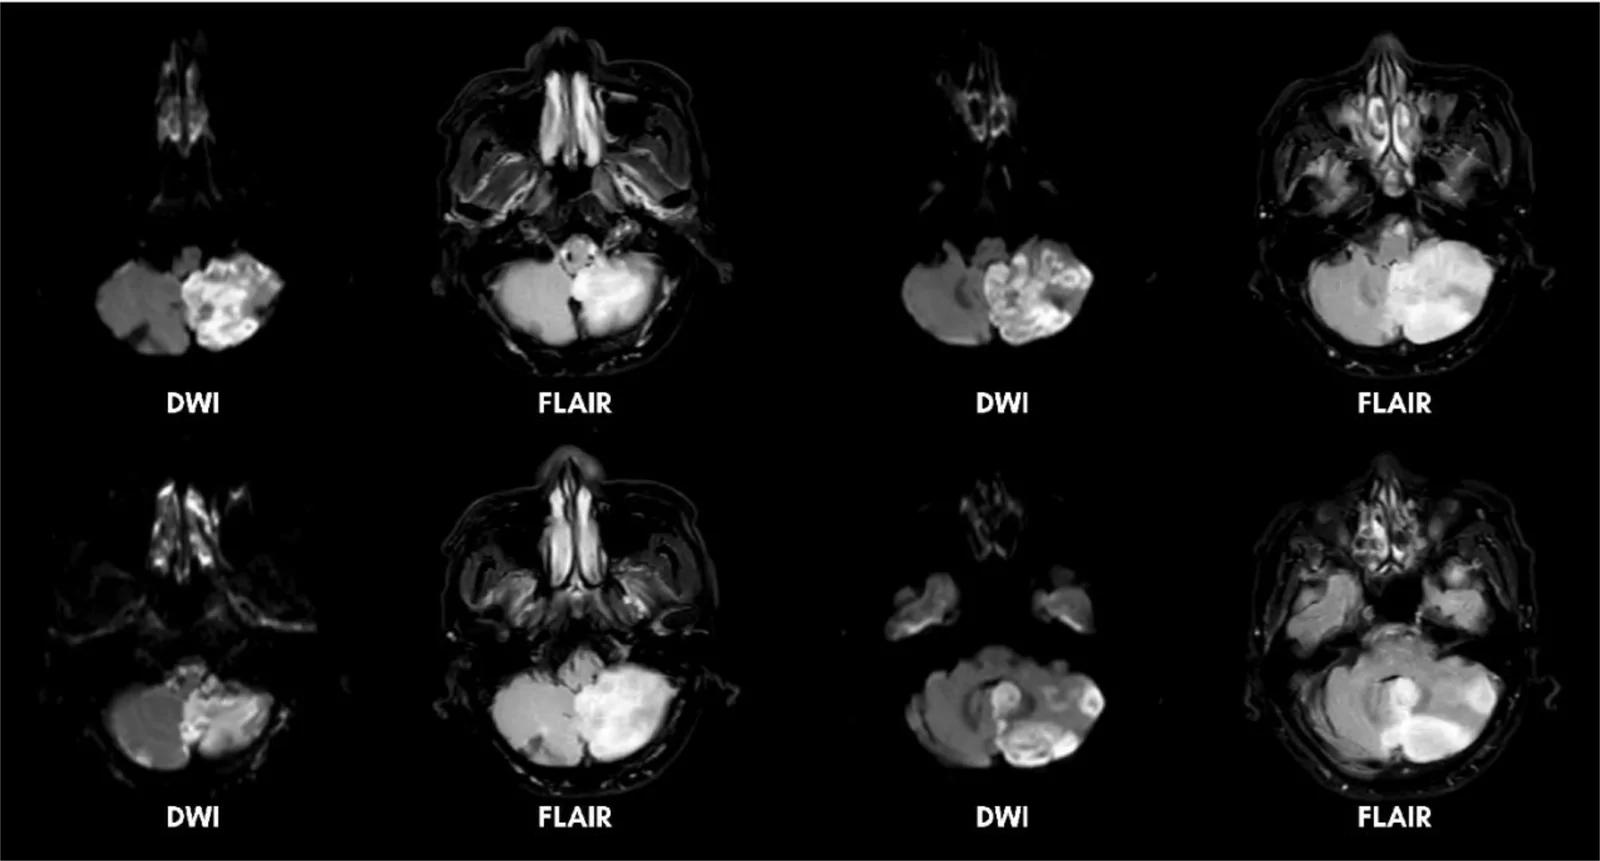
Non-contrast enhanced brain magnetic resonance imaging (MRI) showing a diffusion-restricted lesion on diffusion-weighted imaging (DWI) with hyperintensity and partial hypointensity on T2-weighted fluid-attenuated inversion recovery (T2W FLAIR) in the left cerebellum.
